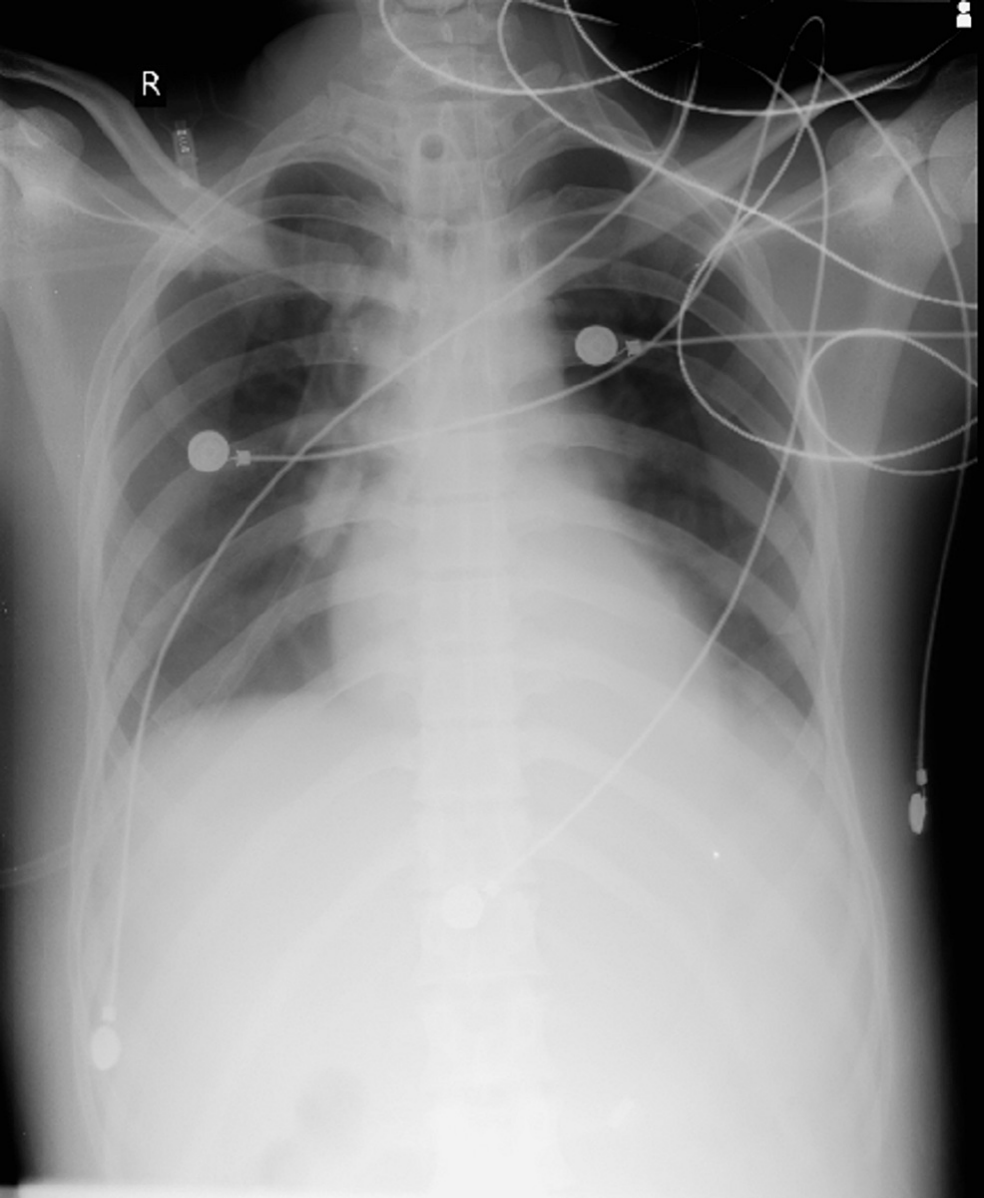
X-ray of January 15, 2023, showing clearing of middle patch
